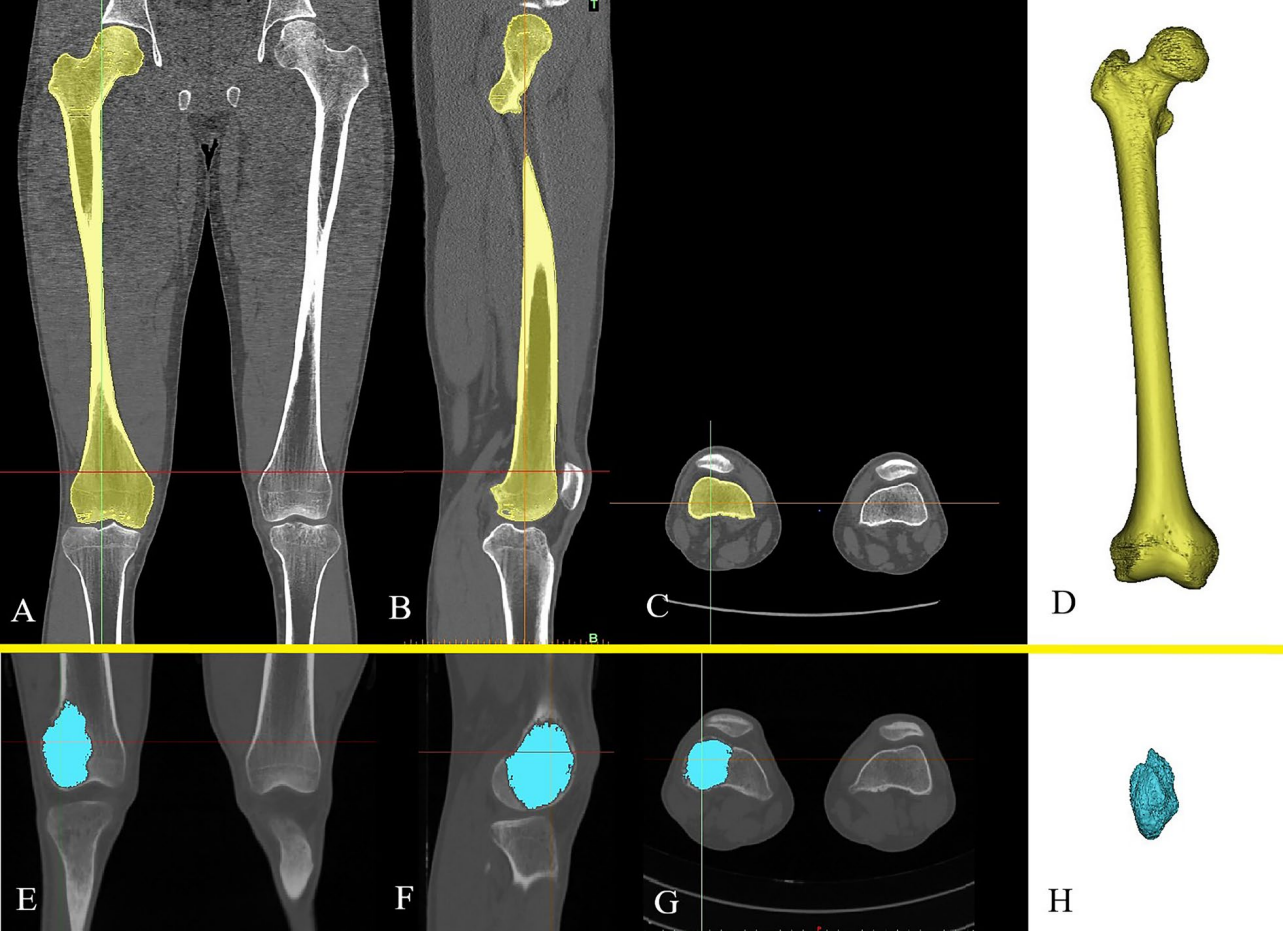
The reconstruction of the normal femur model and the tumor model: A Coronal, B sagittal, and C axial CT images of the normal femur. D The normal femur model. E Coronal, F sagittal, and G axial CT images of the femur with GCTB. H The tumor model
